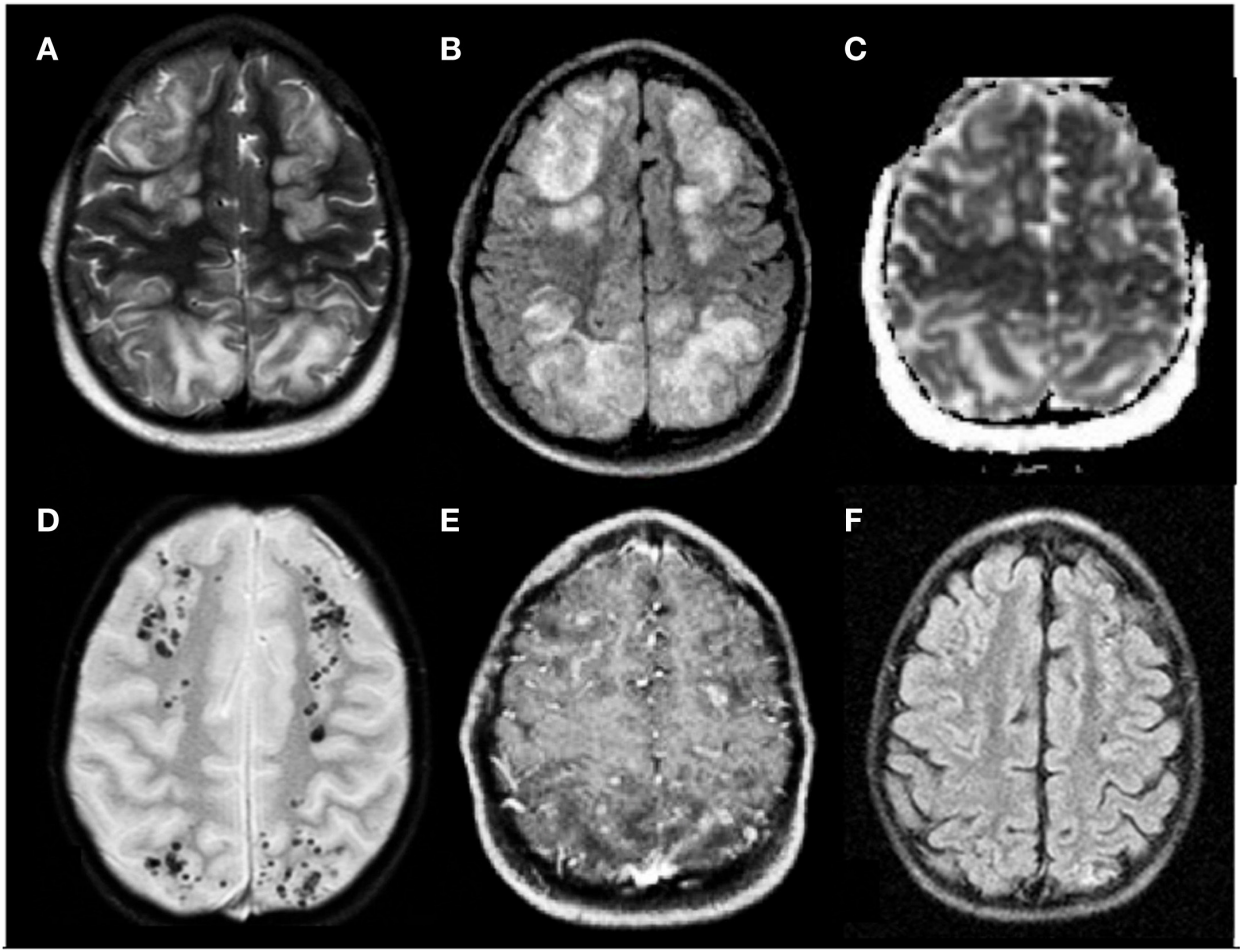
Posterior reversible encephalopathy syndrome in a 5-year-old boy affected by neuroblastoma in treatment with vincristine, etoposide, and carboplatine. (A,B) Axial T2 and FLAIR T2 images, respectively, display iuxtacortical hyperintensities that involve, bilaterally, frontal and parietal parasagittal watershed regions. (C) Diffusion study confirms they represent vasogenic edema. (D,E) Axial T2* and axial T1 post-gadolinium sequences, respectively, reveal microbleeds and faint signs of blood–barrier disruption within the cortex of affected regions. (F) Axial FLAIR T2 image acquired 1 month later shows complete resolution of the vasogenic edema.
